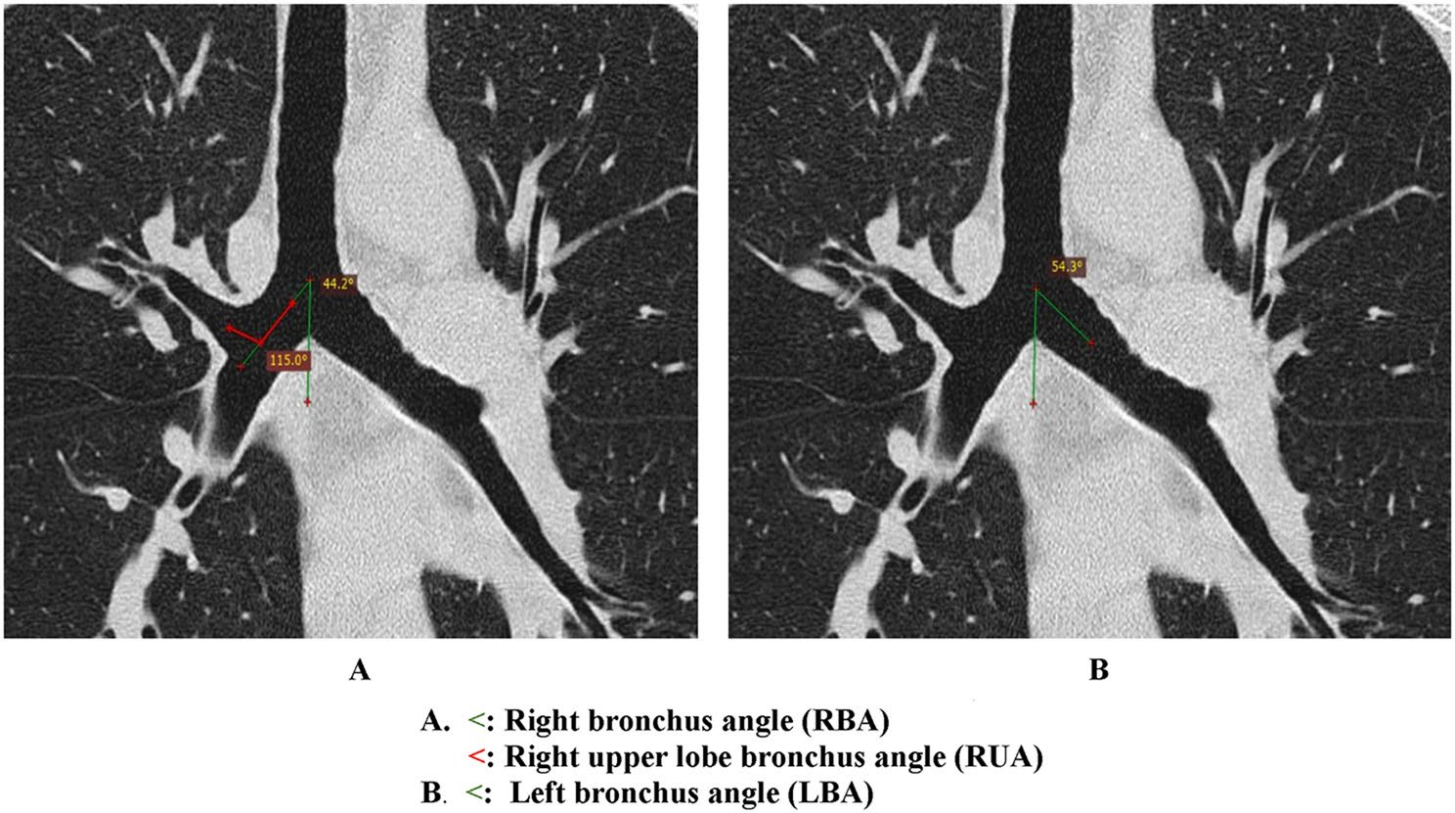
Coronal chest CT reconstructions (lung window) demonstrating bronchial angle measurements
